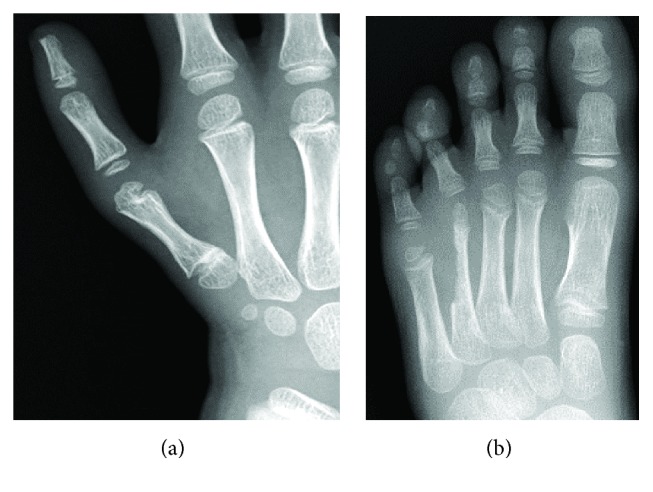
Radiographs of 2 years of follow-up: (a) hand and (b) foot.
